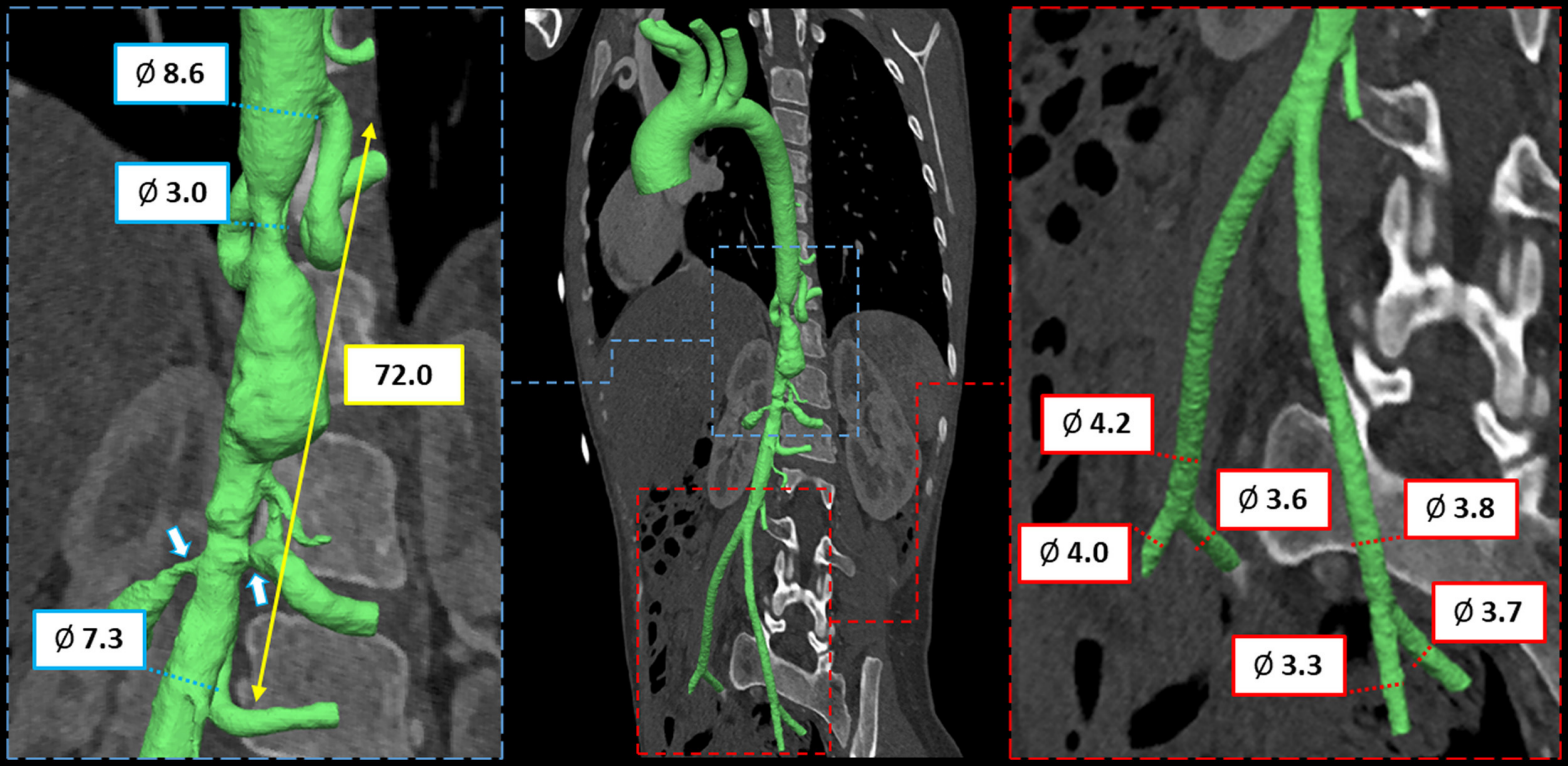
CT imaging and preoperative 3D anatomical model of mid-aortic clinical scenario with virtual measurements, expressed in mm.
